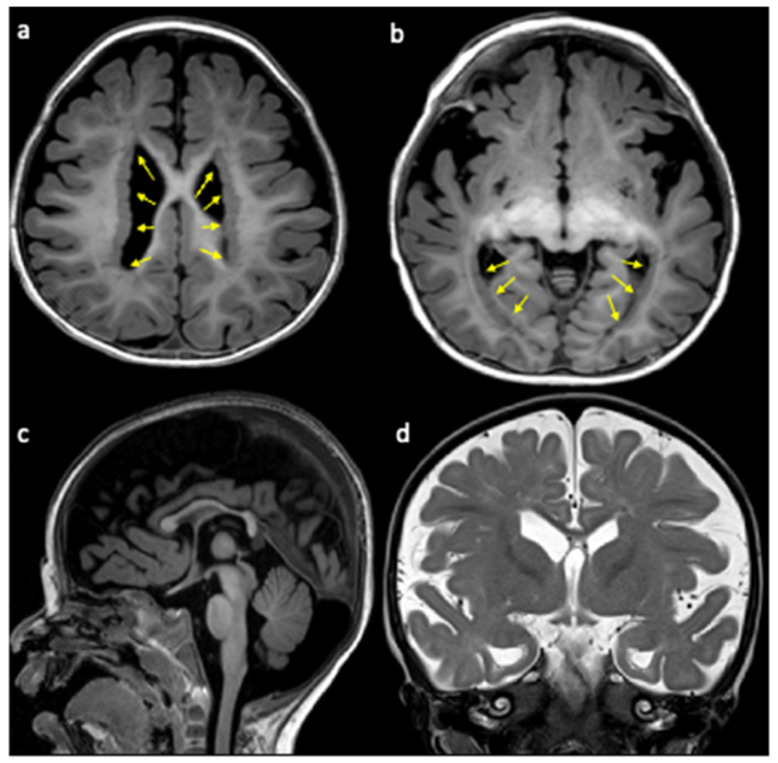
Axial (a,b) and sagittal (c) T1-weighted images and coronal T2-weighted images (d), showing reduced brain trophism, diffuse bilateral periventricular nodular heterotopia (yellow arrows), thin corpus callosum (c), and dysmorphic and enlarged ventricles.
